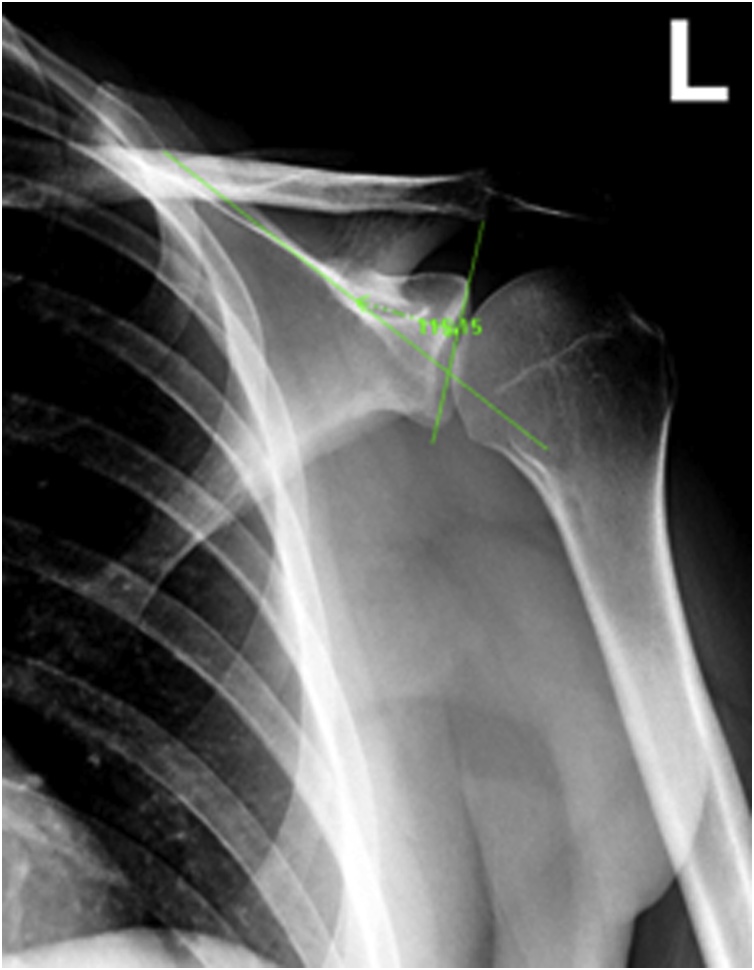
Measurement of the GIA shoulder X-ray in the anteroposterior view. GIA is the angle in the superolateral quadrant.
